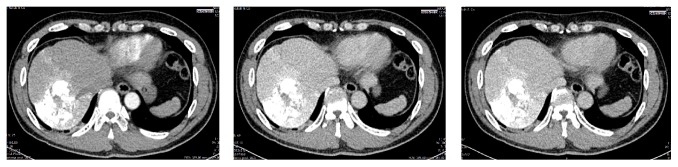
Arterial, venous, and delayed phase of Abdominal CT Scan after first DEB-TACE (January 2013).
